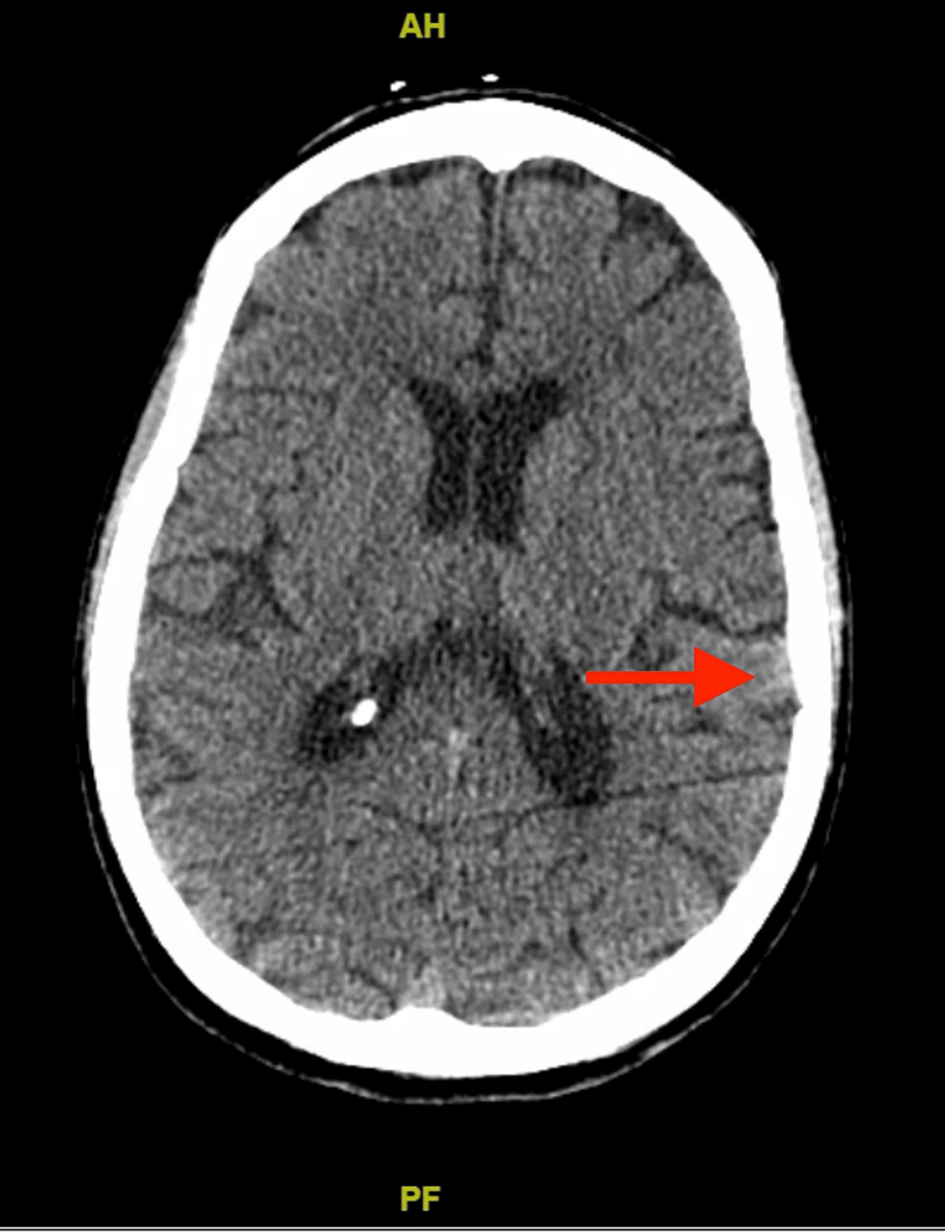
CT scan of the head showing mild chronic microvascular ischemic changes (red arrow).
